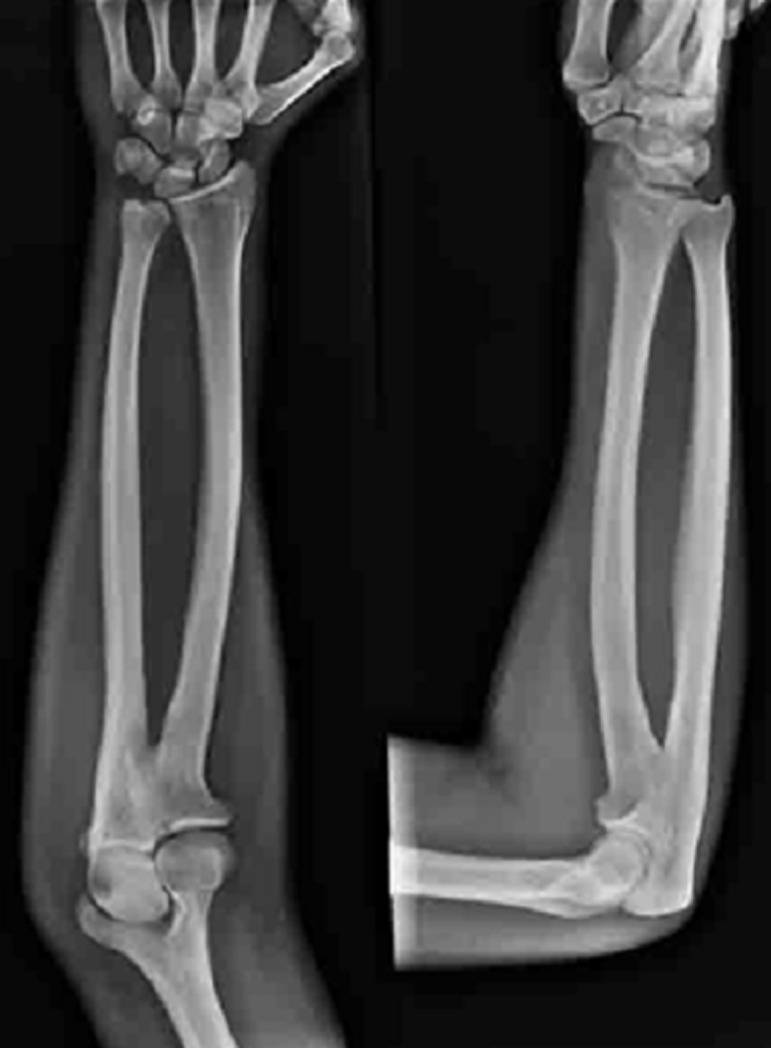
plain x-ray anterior and lateral views of the left elbow joint
